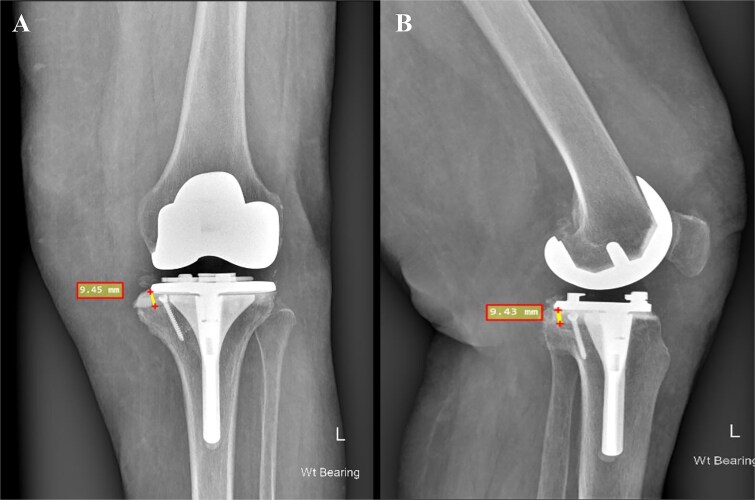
Postoperative face (A) and profile (B) X-rays depicting a medial tibial bone defect ~9.45 mm from the baseplate. The 3.5 mm cancellous screw is indicative of the applied BCSA technique.
